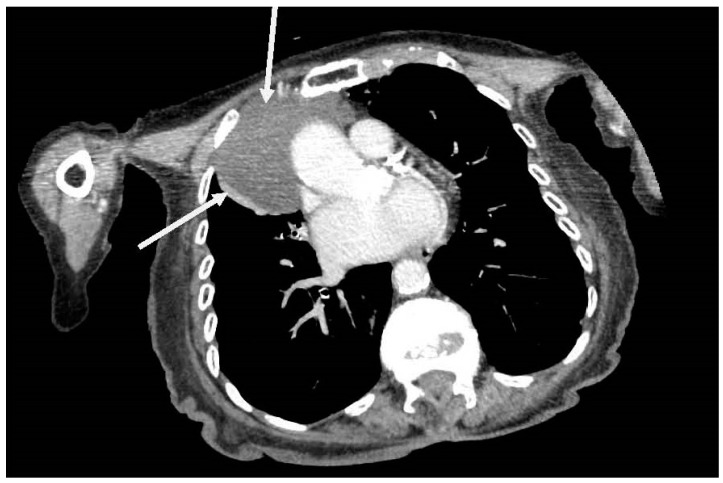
Axial computerised tomography (CT) showing an 8 cm × 5 cm low attenuation right sided soft tissue mass arising from pericardium and abutting the anterior mediastinum and anterior chest wall (arrows).
